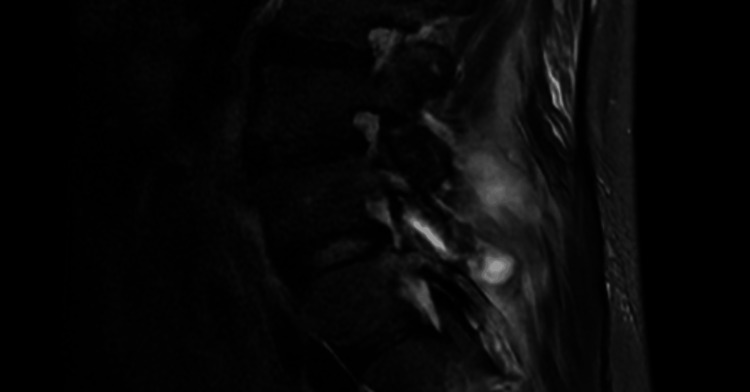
The lumbar spine CT is suspicious for a loculated epidural abscess at the L5 and S1 levels. There is a peripherally enhancing fluid collection in the left erector spinal muscle at the level of L5 and S1, which is suspicious for an abscess.
